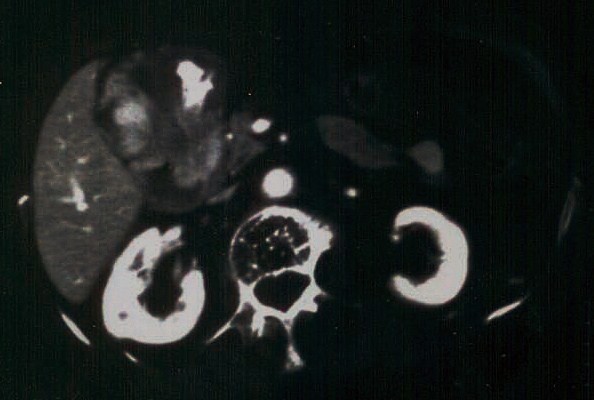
Abdominal CT scan showing an antral tumor and liver metastases.
